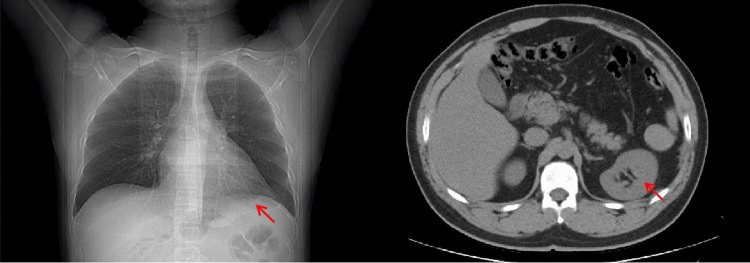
Chest CT at the one-year follow-up. Notable expansion of the left lower lung and the secure fixation of abdominal organs within the abdominal cavity (arrow).
